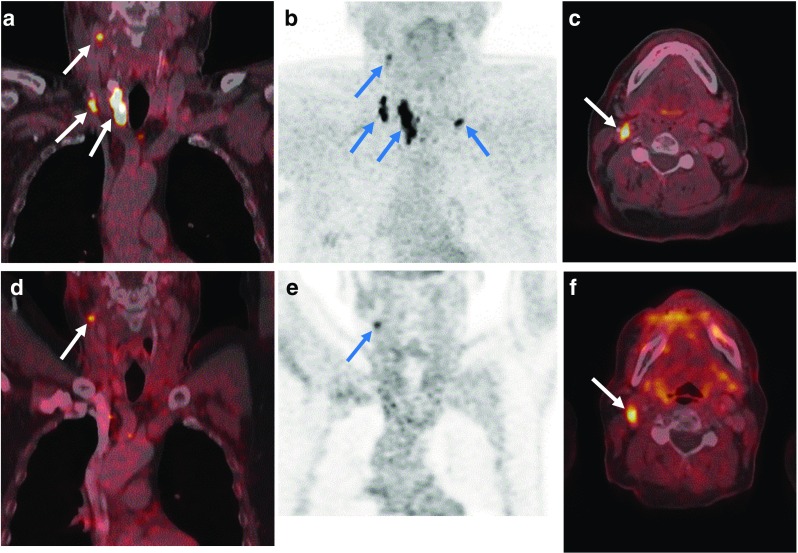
A 61-year-old male patient with persistently elevated basal calcitonin levels postoperatively. a–c Preoperative [^18^F]DOPA PET/CT images show increased DOPA uptake in the primary tumour in the right thyroid lobe as well as multiple bilateral DOPA-positive cervical lymph nodes (levels II, III, IV and VI). d–f Postoperative [^18^F]DOPA PET/CT images in the same patient 2 months after total thyroidectomy with central and bilateral neck dissection, show a residual lymph node with focal DOPA uptake in the right upper cervical region (level II)
